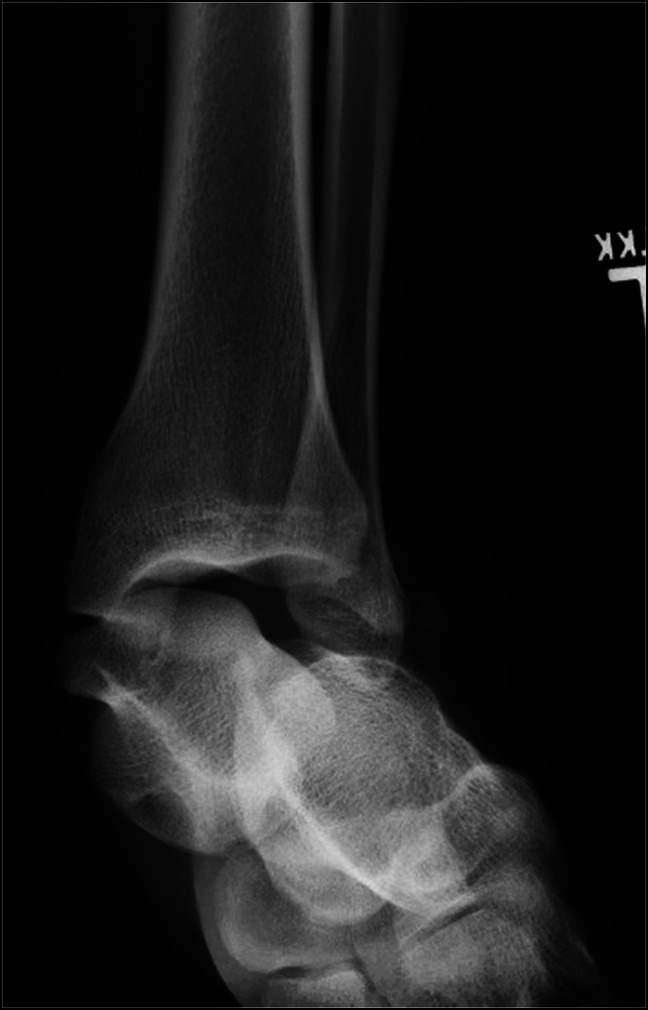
Prereduction anteroposterior ankle radiograph showing medial dislocation of the talus.
